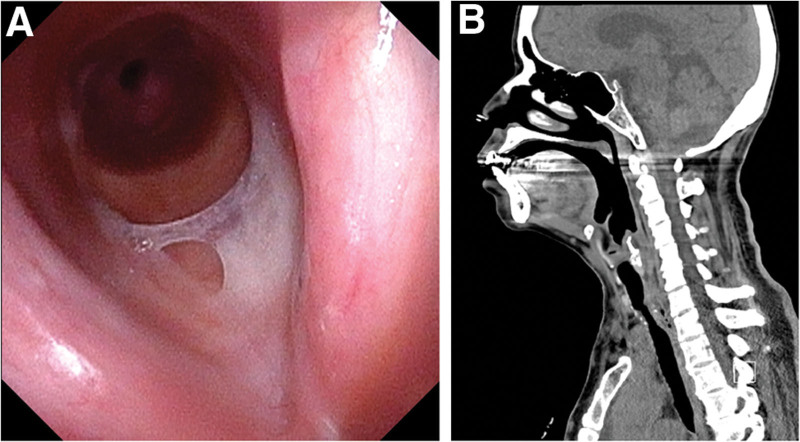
(A) Preoperative fiberoptic laryngoscopy image showing Mayer–Cotton grade 3 subglottic stenosis. (B) Preoperative neck computed tomography (sagittal view) showing tracheal stenosis.
